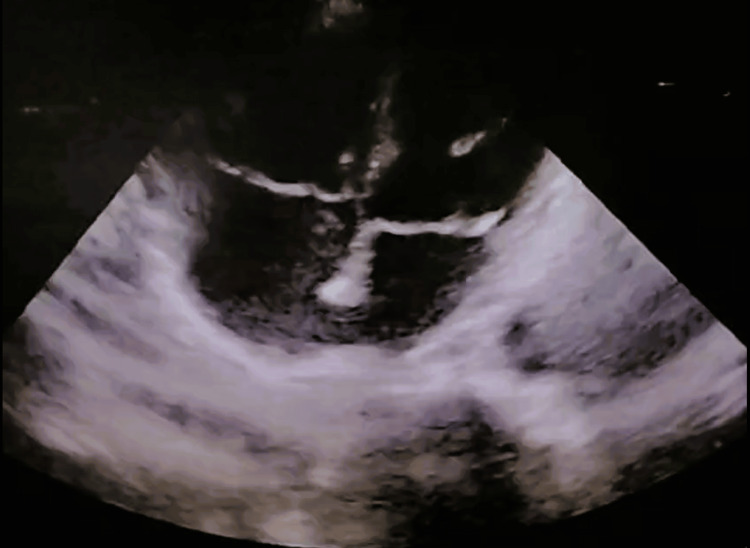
Apical four-chamber view showing a dilated right atrium and right ventricle
